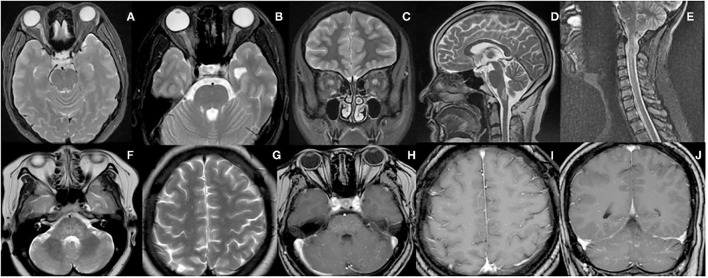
MRI features of the representative patients. (A) A 40-year-old woman presented with acute vision loss of the right eye, and MRI scans revealed hyperintense lesion in the right optic nerve on T2-weighted images. (B,C) A 26-year-old woman presented with acute vision loss of both eyes, and MRI scans revealed hyperintense lesions in the bilateral optic nerves on axial and coronal T2-weighted images. (D) A 45-year-old woman presented with persistent nausea and vomiting, and MRI scans revealed a hyperintense lesion in the medulla on sagittal T2-weighted images. (E) A 44-year-old woman presented with numbness in her right upper extremity, and a hyperintense lesion in the cervical spinal cord was shown on sagittal T2-weighted images. (F–J) In the CLIPPERS-form case, extensive lesions were shown in the pons, pontibrachium, cerebellum, and hemispheres on T2-weighted images and after gadolinium-enhanced T1-weighted images, and a typical peppering sign was seen in both supratentorial and infratentorial [T2 scans are F&G while T1 gadolinium contrast scans are (H–J)].
